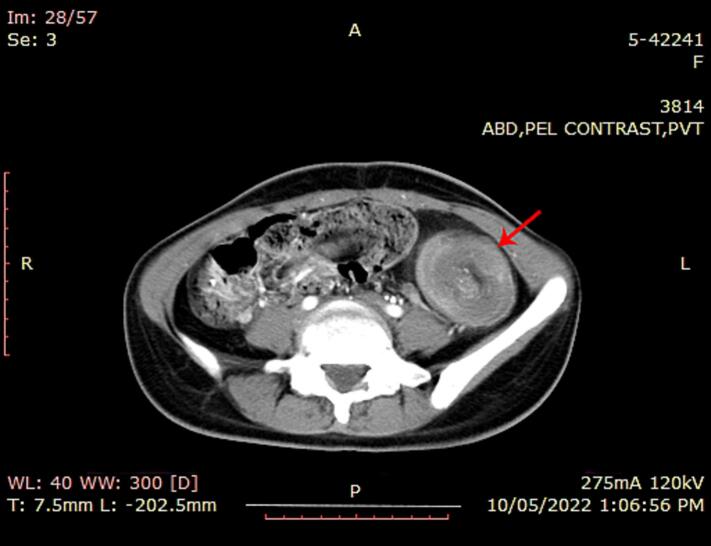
CT imaging with contrast showing concentric mass in left hemiabdomen with “bowel within bowel” appearance.
